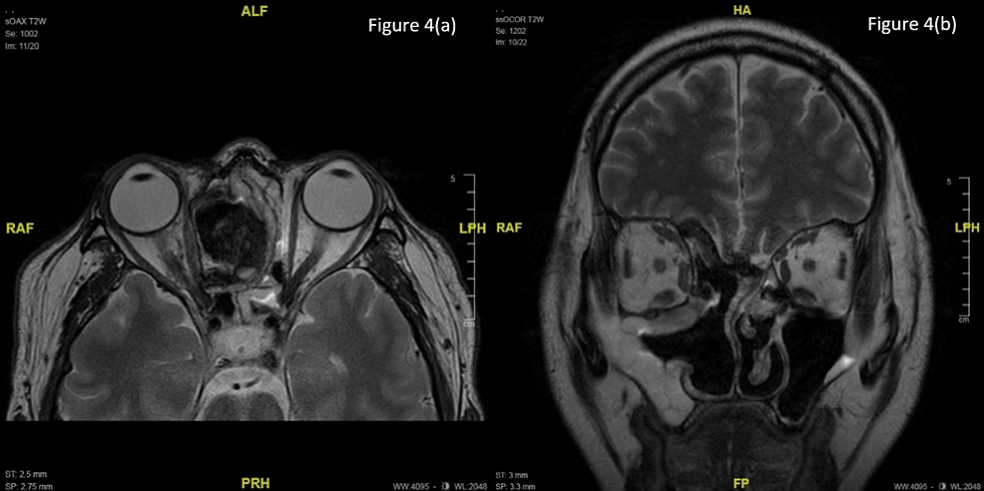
Postoperative axial (a) and coronal (b) T2-weighted MRI image of the brain showing no residual lesion with the resolution of right eye proptosis with symmetrical optic nerves and preservation of extraocular muscles.
